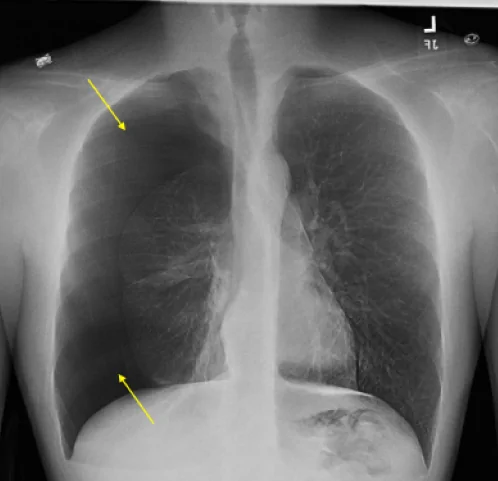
Chest radiograph demonstrating large right-sided pneumothorax.
